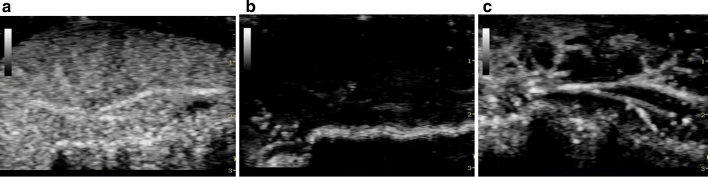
Contrast-enhanced ultrasound images of the liver in the TUS + MB + HT group. The treated liver lobe showed homogeneous enhancement before treatment (a); Liver perfusion was temporarily blocked after MEUS (b), and partially recovered after HT (c)
